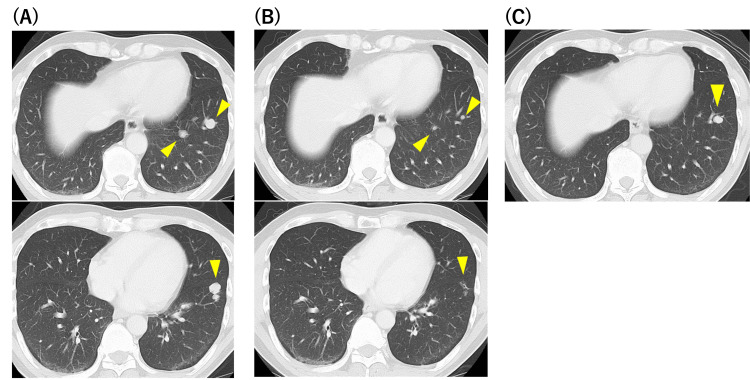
Chest CT images revealed the clinical course of pulmonary metastases. (A) Chest CT revealed multiple pulmonary metastases five months after the surgery; (B) Multiple pulmonary metastases shrank with chemotherapy; (C) Single pulmonary nodule appeared 15 months after the chemotherapy.
